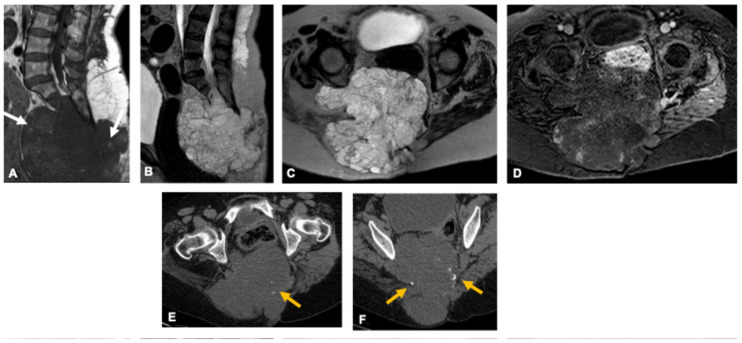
Chordoma in a 62-year-old woman. Sagittal T2-weighted MR image (A) and sagittal T1-weighted image (B) show an extensive osteolytic lesion centered in the sacrococcygeal region with cortical destruction and a large lobulated extraosseous component extending anteriorly into the pelvis and posteriorly into the spinal canal. The lesion exhibits high T2 signal intensity with internal hypointense septations and heterogeneous post-contrast enhancement (D). On T1-weighted imaging, focal areas of high signal (arrows in (A)) correspond to intratumoral hemorrhage or proteinaceous material. CT images (C,E,F) demonstrate an expansile destructive mass with amorphous peripheral calcifications (yellow arrows), representing residual bone sequestra rather than dystrophic calcification.
